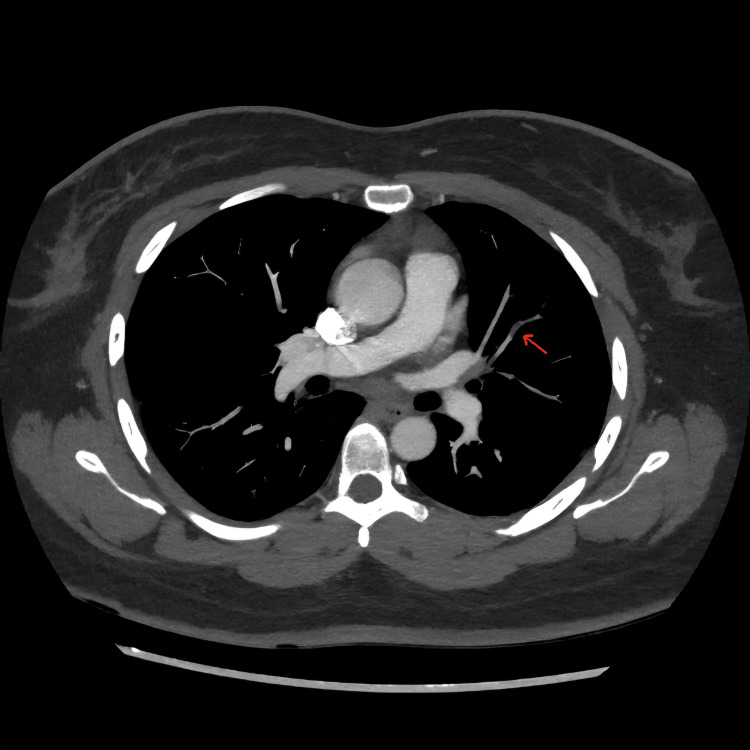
Axial CT pulmonary angiography showing a subtle intraluminal filling defect in a left lobe sub-segmental pulmonary artery. The arrow points to a very subtle, low-attenuation, and hypodense intraluminal filling defect in a left lobe sub-segmental pulmonary artery. The hypodensity is maximum at the genu of the pulmonary artery, most likely attributed to a mild fat embolism.
